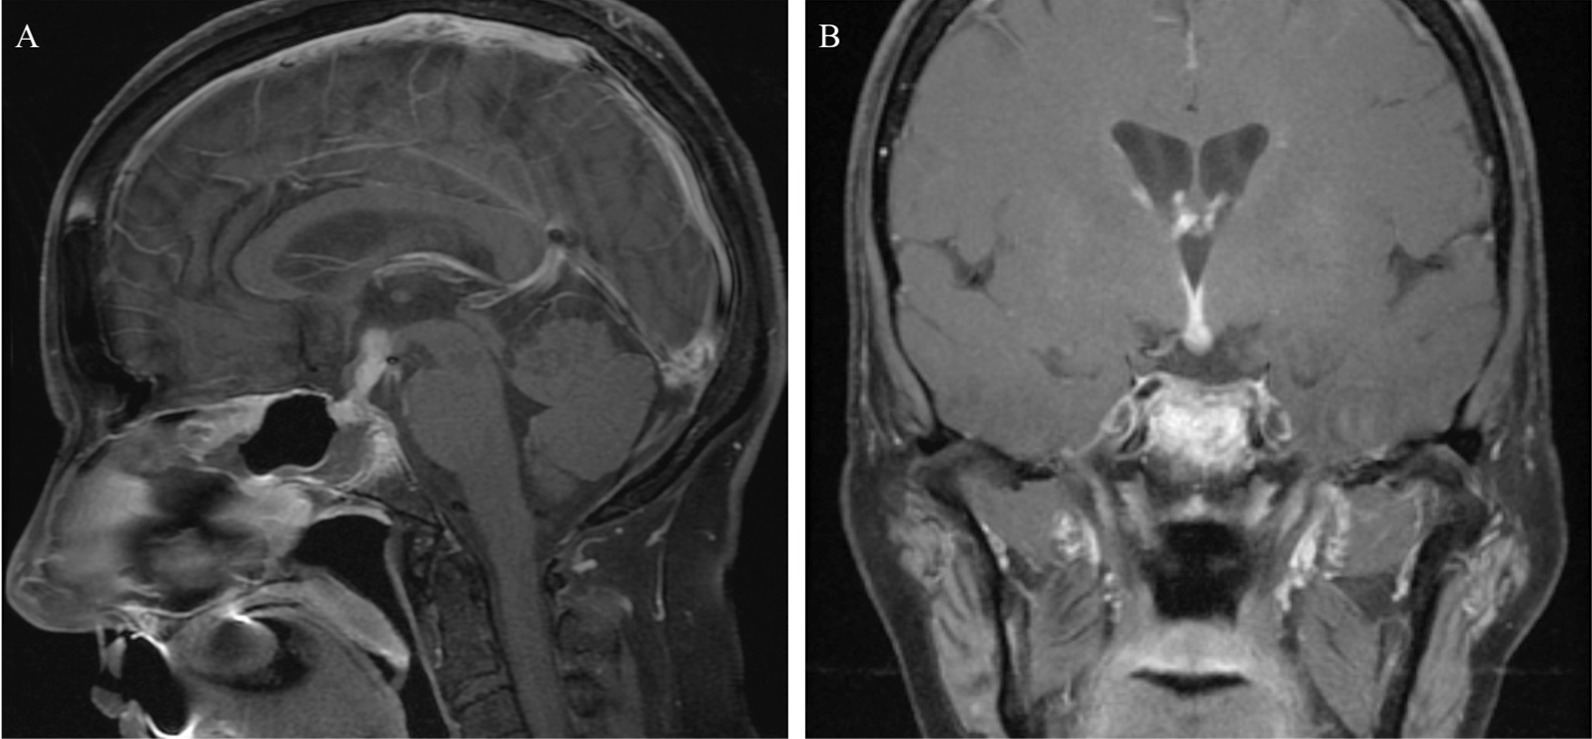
MRI brain with and without gadolinium 5 days post-biopsy and high-dose steroids. Sagittal T1 post-gadolinium (A). Coronal T1 post-gadolinium (B)
